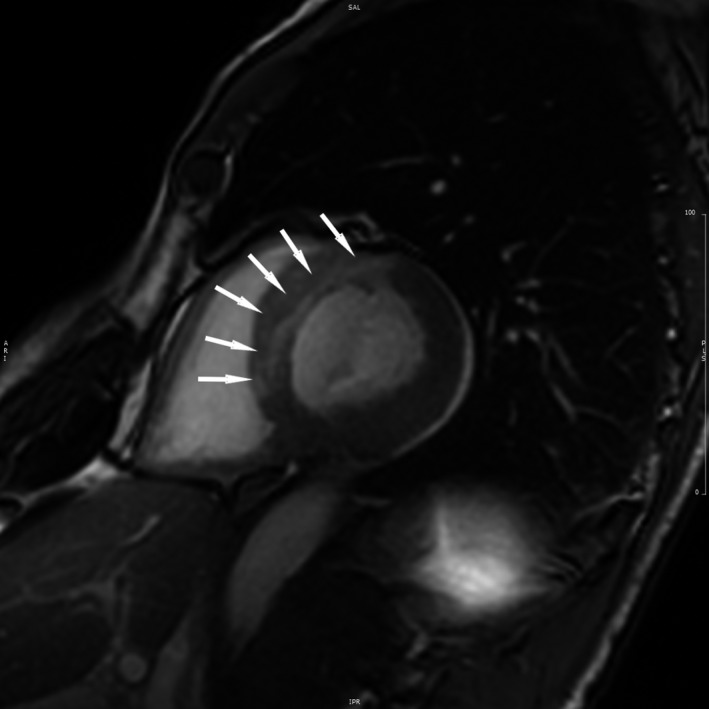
MRI scan showing large anteroseptal myocardial infarction (white arrows)
